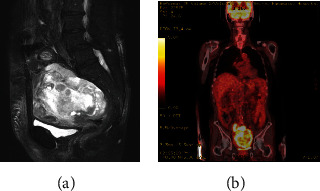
Tumor imaging. (a) Pelvic MRI on sagittal T2WI. A 15 cm tumor showing inhomogeneous high signal intensity (arrow). (b) PET-CT coronal imaging. Increased accumulation of 18F fluorodeoxyglucose in the pelvic tumor.
